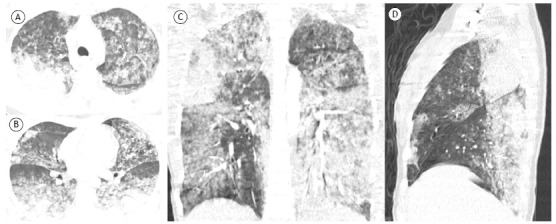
Axial chest CT images (in A and B), as well as coronal and sagittal chest CT images (in C and D, respectively), showing areas of consolidation and ground-glass attenuation distributed diffusely throughout the lungs.
